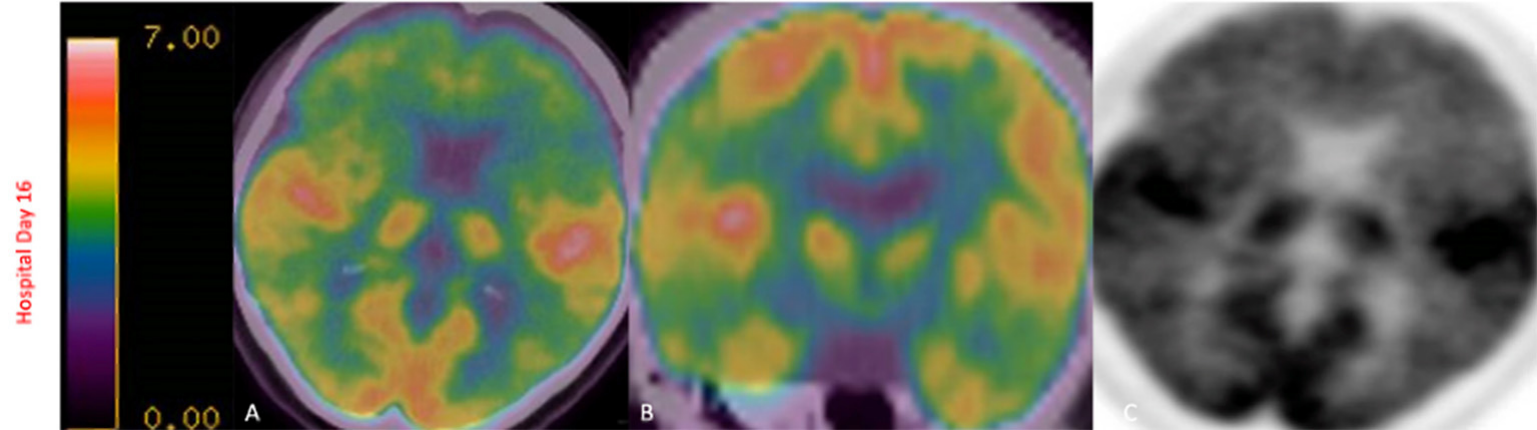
FDG-PET scan on hospital day 16 revealed decreased focal uptake in both globus pallidus, diffuse supratentorial hypometabolism with relatively increased tracer uptake in both anteromedial temporal lobes, including the hippocampal formations, parahippocampal gyrus, and amygdala, findings that have been described in limbic encephalitis (A–C).
